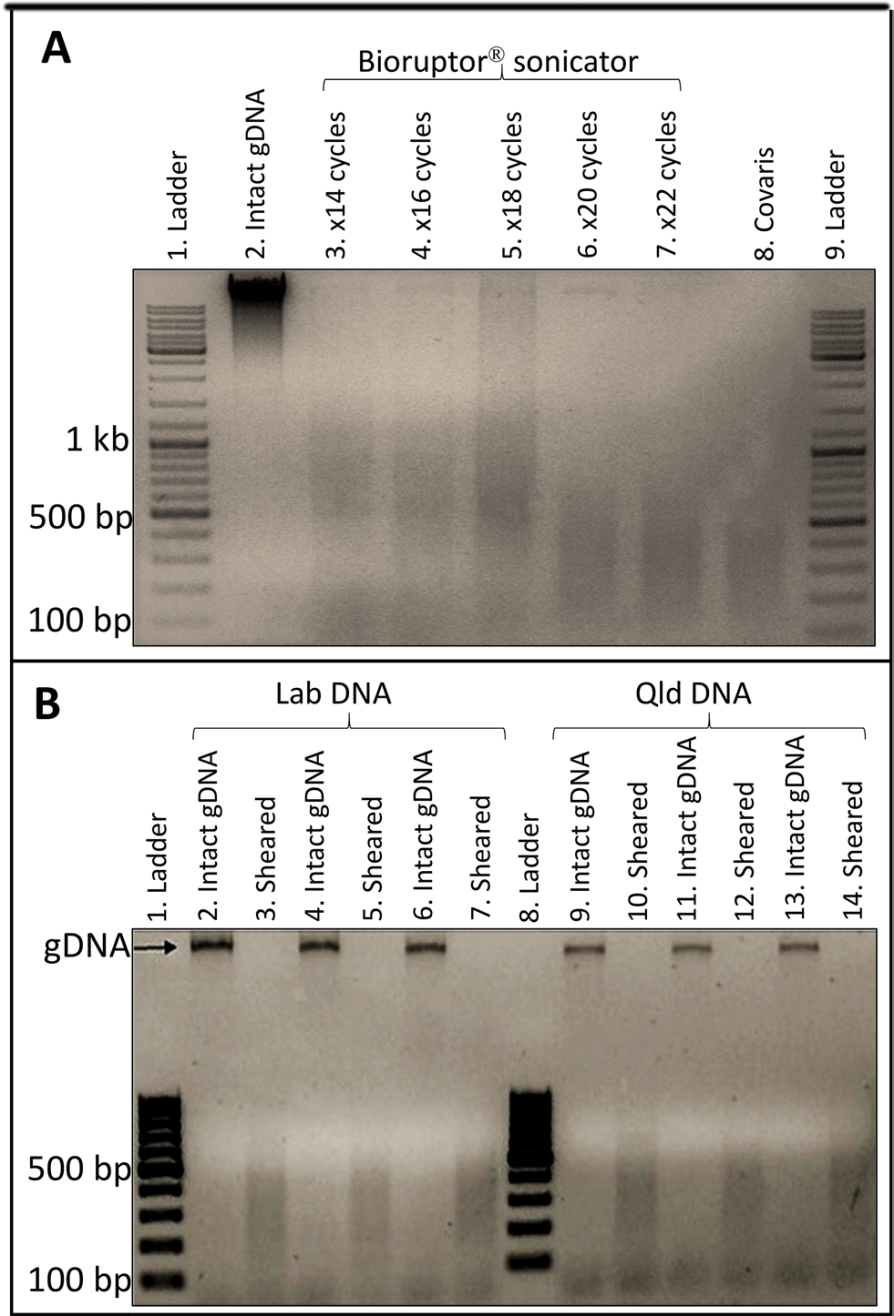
Comparison of DNA shearing efficiency using Bioruptor sonicator and Covaris ultrasonicator. a Optimisation of the number of sonication cycles required with Bioruptor^®^ sonicator to fragment gDNA compared to Covaris ultrasonicator. The desired fragmentation range (100–800 bp) achieved with 20 or 22 cycles using Biorupter whereas with Covaris the 150 bp instrument setting was sufficient. b Three biological replicates of Lab and Qld intact gDNA sheared with Covaris ultrasonicator using the 150 bp instrument setting to generate reproducible fragment range in all replicates
